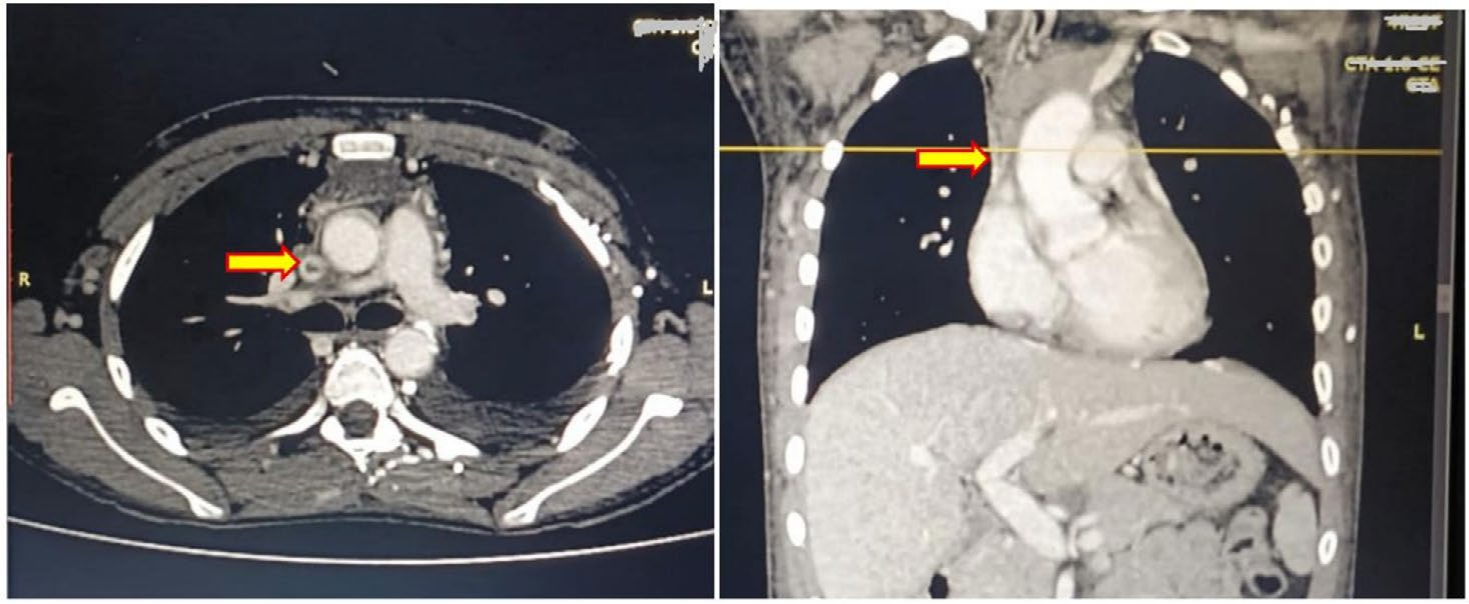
CT imaging showing a large thrombus in the superior vena cava. A large filling defect (thrombus) is visible within the lumen of the superior vena cava (SVC), as indicated by the arrow.
